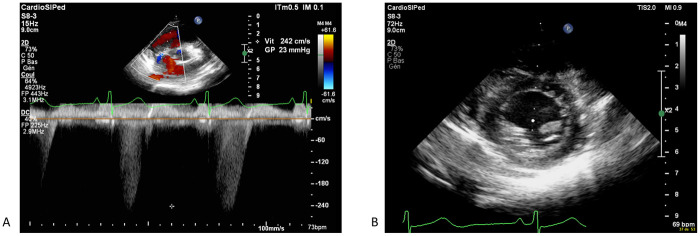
Echocardiography showing a significant improvement after 48 h of treatment, with regression of tricupsid regurgitation and complete normalizazion of right venticular pressure (A) with normal motion of the interventicular septum (B).
